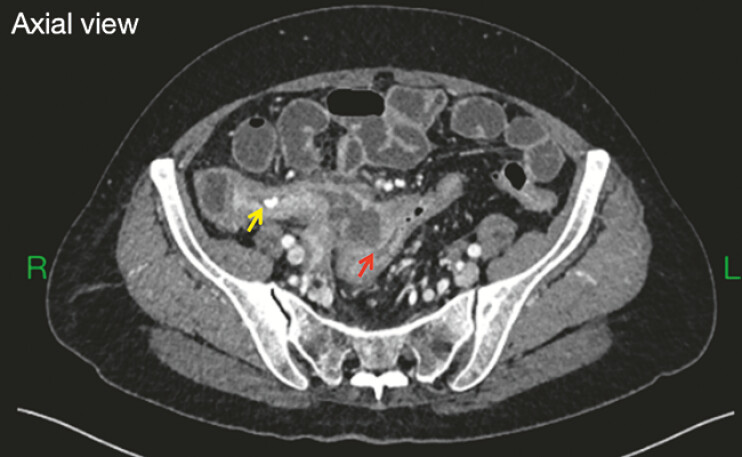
Computed tomography scan showed appendicitis accompanied by a fecalith (yellow arrow) and a local abscess, suggesting possible sigmoid fistula (red arrow).
